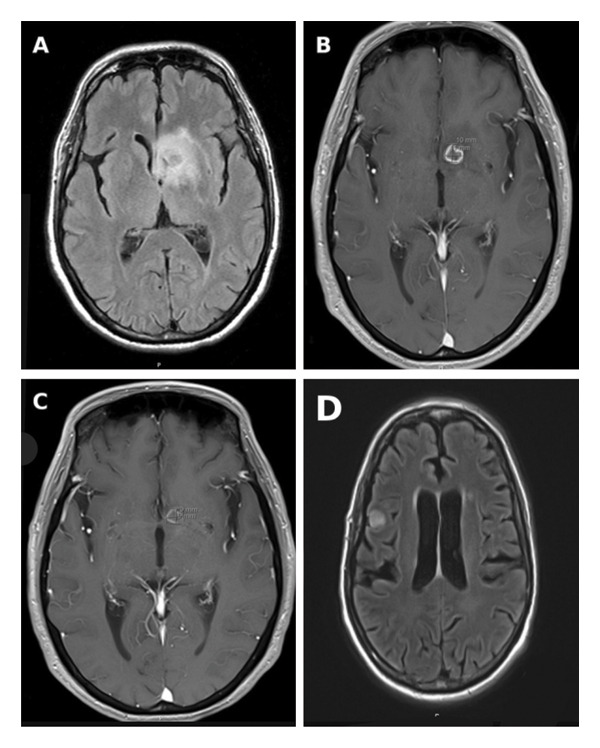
Initial MRI brain (A–D). (A) Axial FLAIR image demonstrating a hyperintense lesion centered in the left basal ganglia with surrounding vasogenic edema. (B) Axial postcontrast T1‐weighted image demonstrating rim enhancement of the basal ganglia lesion. (C) Axial postcontrast T1‐weighted image at a slightly inferior level demonstrating persistent enhancement of the lesion. (D) Axial postcontrast T1‐weighted image demonstrating a second rim‐enhancing lesion in the right frontal lobe, consistent with multifocal disease.
